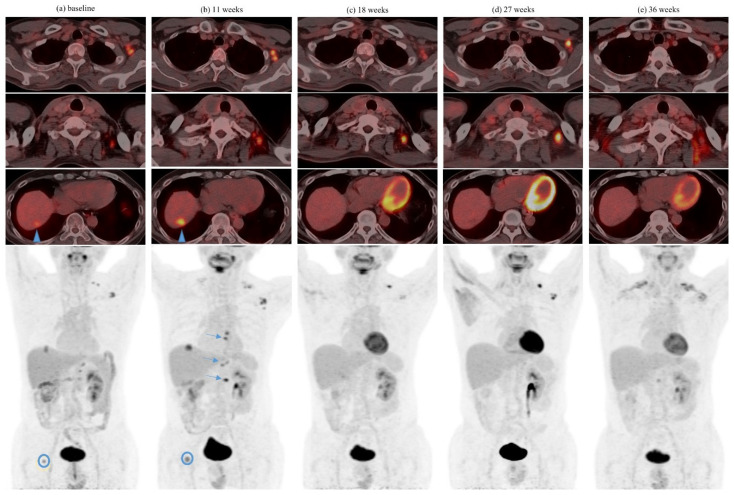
Serial pseudoprogression (inter- and intra-tumoral) in a 54 year-old gentleman on ipilimumab-nivolumab for metastatic renal cell carcinoma. (a) Baseline FDG positron emission tomography (PET)/CT demonstrates FDG-avid left supraclavicular and axillary lymphadenopathy, an FDG-avid liver dome metastasis (arrowhead), as well as a focal FDG-avid metastatic deposit in the right femoral head (blue circle). (b) Assessment at 11 weeks showing apparent disease progression with an increase in FDG-avidity of left axillary adenopathy, hepatic metastasis, and right femoral head metastasis as well as new FDG-avid mediastinal and upper abdominal adenopathy (arrows on maximal intensity projection (MIP) image). (c) Assessment at 18 weeks demonstrates metabolic resolution of mediastinal and upper abdominal lymphadenopathy, hepatic, and right femoral head metastasis. There is an interval decrease in FDG-avidity of left axillary adenopathy but an increase in left supraclavicular adenopathy. (d) Reassessment at 27 weeks showing further increase in FDG-avidity of left supraclavicular adenopathy and a recurrent increase in FDG-avidity in the axillary adenopathy. (e) Reassessment at 36 weeks showing decrease in FDG-avidity of left axillary lymphadenopathy and metabolic resolution of left supraclavicular lymphadenopathy. Physiological brown fat FDG uptake is seen in the supraclavicular regions.
